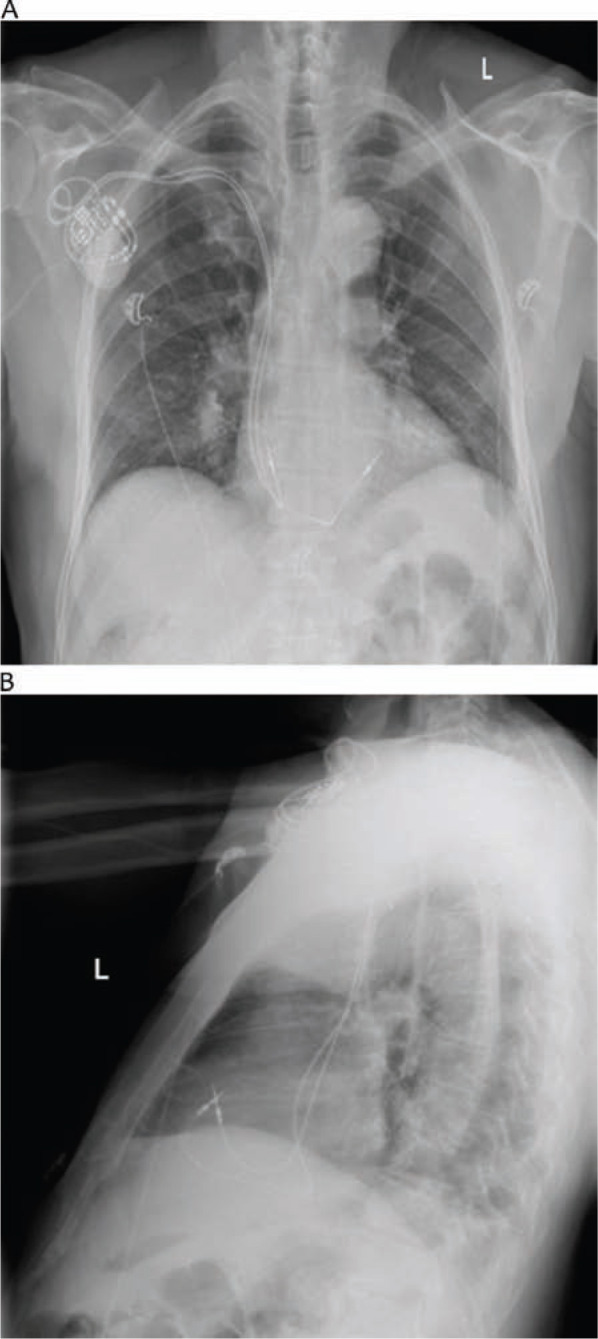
Chest radiograph of a dual-chamber pacemaker implanted with right ventricular lead in the right ventricular outflow tract. A: Posterioranterior view. B: Lateral view.
